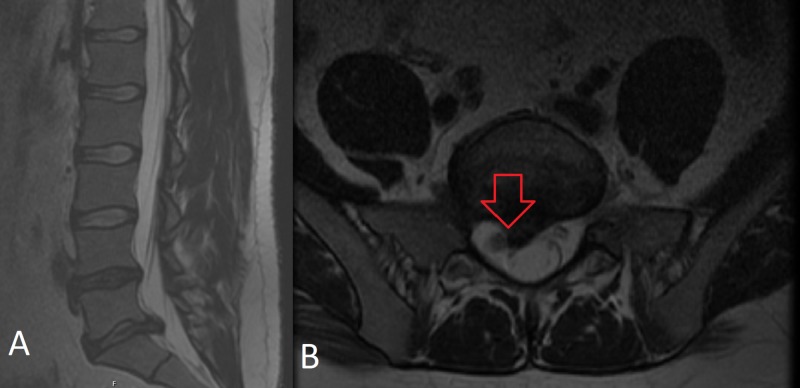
A. T2 sagittal MRI of the lumbar spine w/o contrast B. Axial MRI of the lumbar spine w/o contrast A. Sagittal T2 w/o contrast MRI lumbar spine shows a large 9 mm L5/S1 paracentral disc protrusion with mass effect on the thecal sac. B. Axial T2 w/o contrast MRI lumbar spine; the same patient shows compression of the right exiting S1 nerve root, which has caused this patient to experience right S1 radiculopathy.
